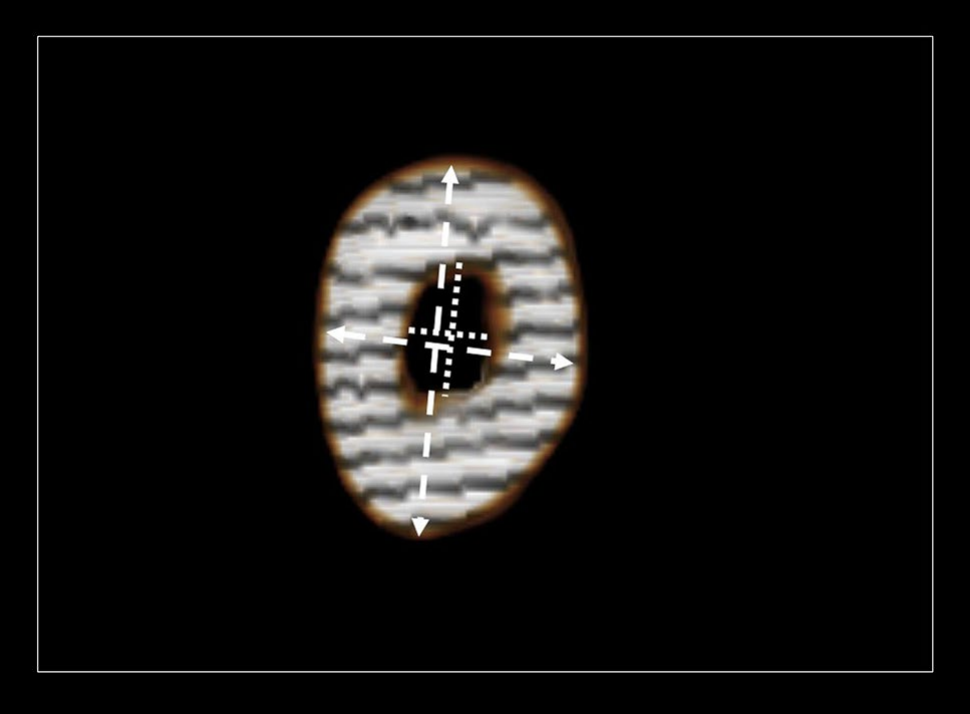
Computed tomography 3D Reconstruction coronal section at isthmus level of the fifth metatarsal bone. The bone’s bowing was defined as the narrowest area of the medullary canal and was used as reference point for isthmus’s measurements. HDI: horizontal diameter of the isthmus (horizontal white dotted line), VDI: vertical diameter of the isthmus (vertical white dotted line), VODI: vertical outer diameter of the isthmus (vertical white double-headed arrow), HODI: horizontal outer diameter of the isthmus (horizontal white double-headed arrow)
